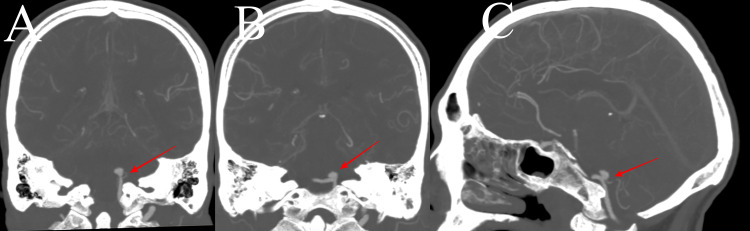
Computed tomography angiogram of the brain Coronal sections showing the origin of the aneurysm from the left posterior inferior cerebellar artery (PICA) takeoff (A, red arrow) and the location in relation to the occipital condyle (B, red arrow). (C) shows a sagittal section of the aneurysm in relation to the left vertebral artery and PICA (red arrow). The course of PICA can be appreciated.
